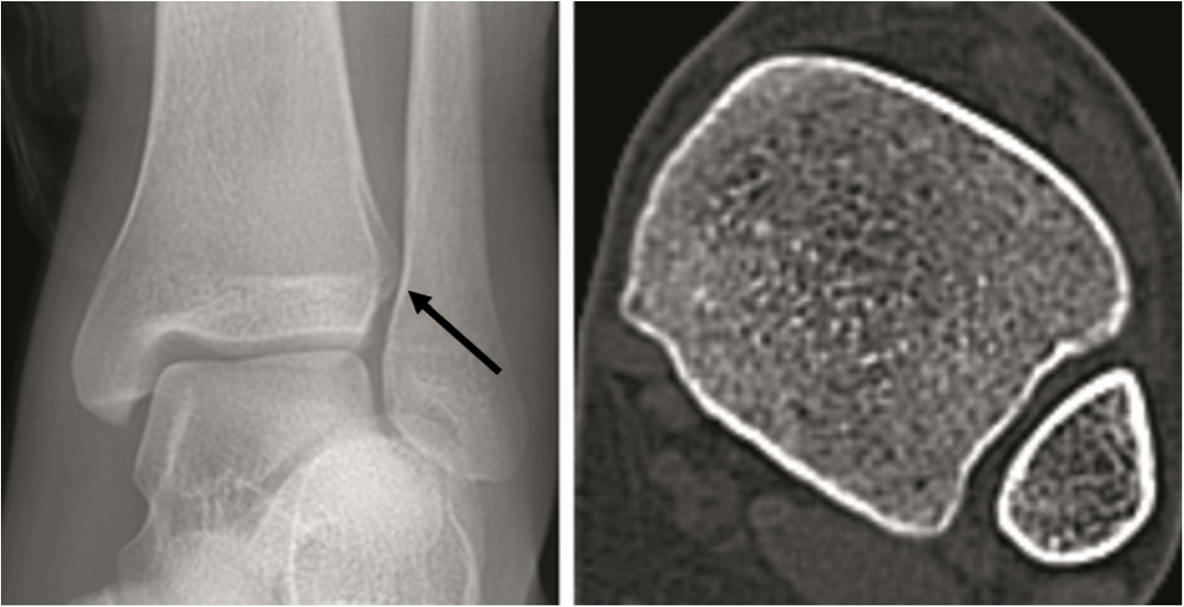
X-ray and CT scans of the DTS with a separated left ankle joint and b normal left ankle joint. The position indicated by the black tip show the lack of tibiofibular overlap
